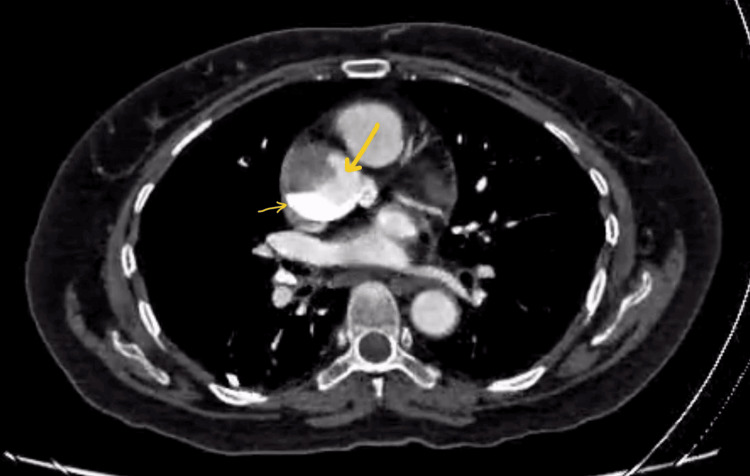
CT aortogram confirming ascending aortic dissection. Small arrow indicates the contrast in dissection flap; Bold arrow indicates the true lumen of ascending aorta.
